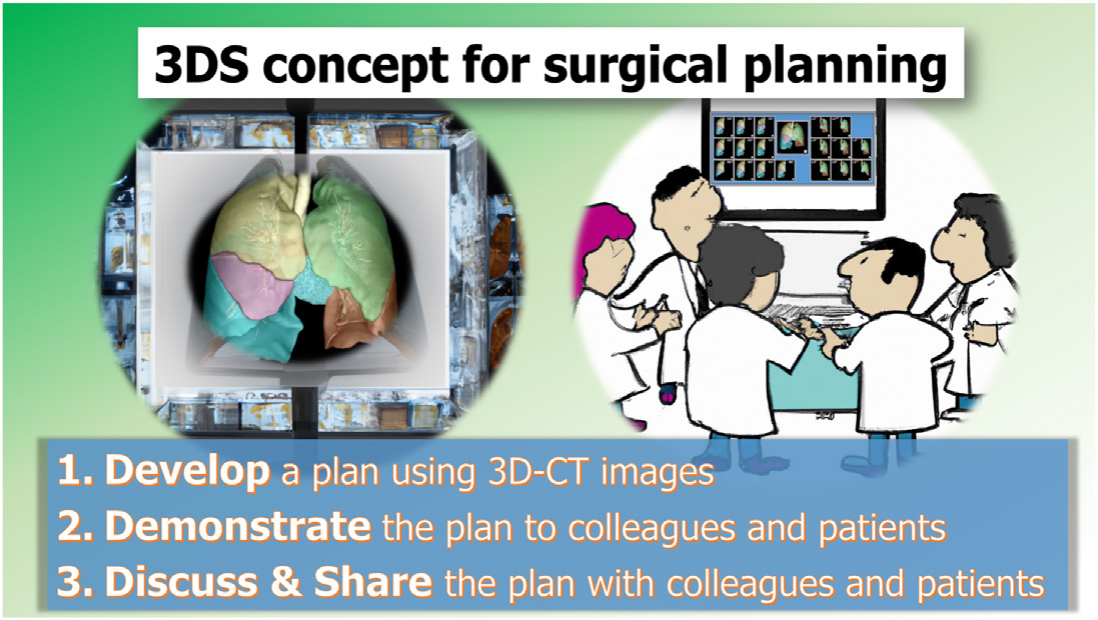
The development, demonstration, discussion, and sharing (3DS) concept for surgical planning. (3D-CT, 3-dimensional computed tomography.)
